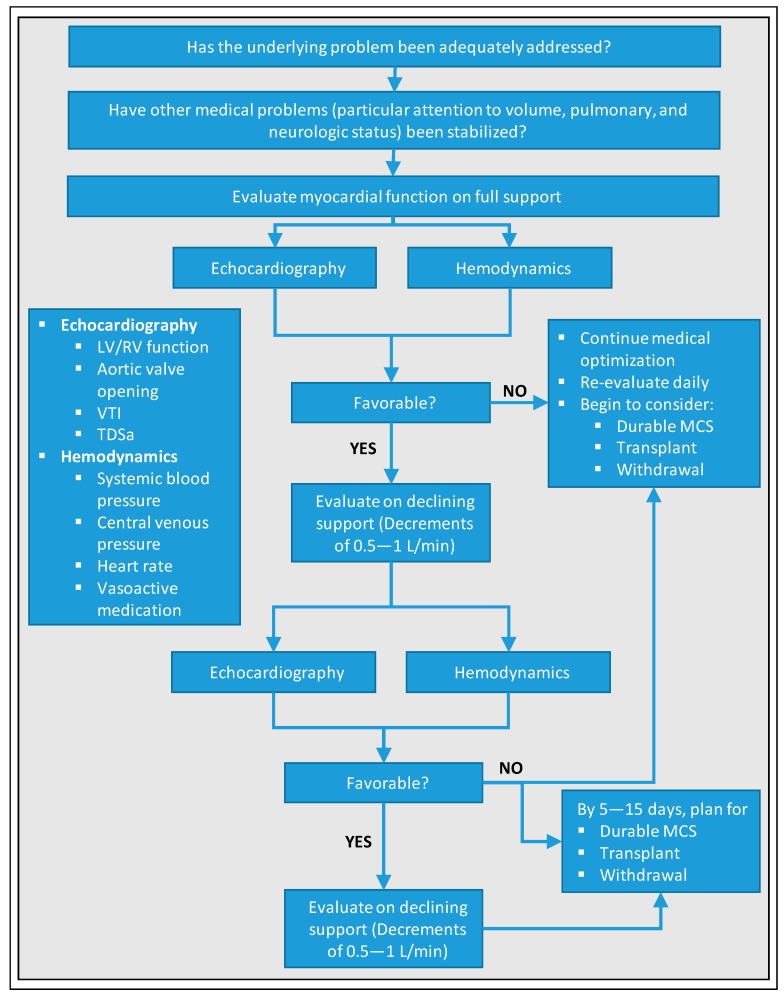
Standardized protocol for weaning from VA-ECMO according to Eckman et al. [10]. LV, left ventricle; RV, right ventricle; VTI, velocity-time integral; TDSa, tissue Doppler lateral mitral annulus peak systolic velocity; MCS, mechanical circulatory support.
